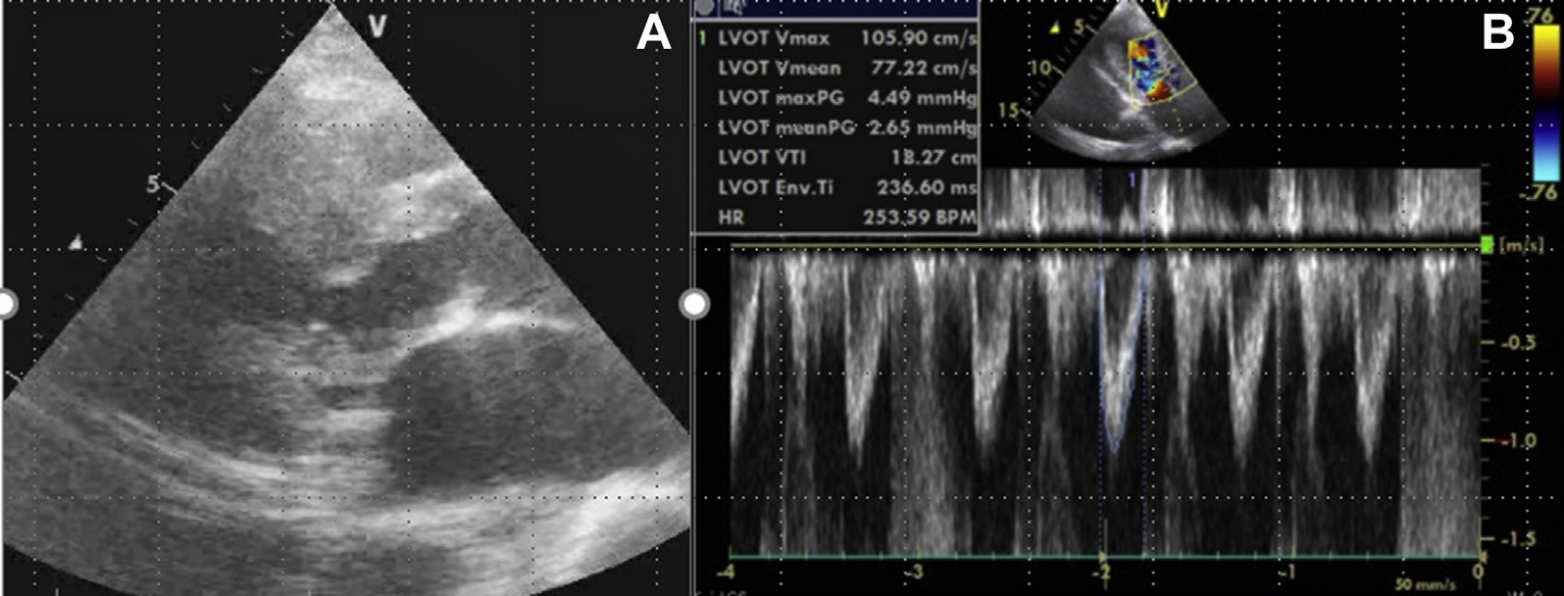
Transthoracic Echocardiography Findings After Percutaneous Transluminal Septal Myocardial Ablation (A) Decrease in left ventricular outflow obstruction after percutaneous transcatheter septal myocardial ablation, and (B) the accelerated blood flow of the left ventricular outflow tract (LVOT) decreased to approximately 1.1 m/s on transthoracic echocardiography without the support of extracorporeal membrane oxygenation after percutaneous transcatheter septal myocardial ablation.
